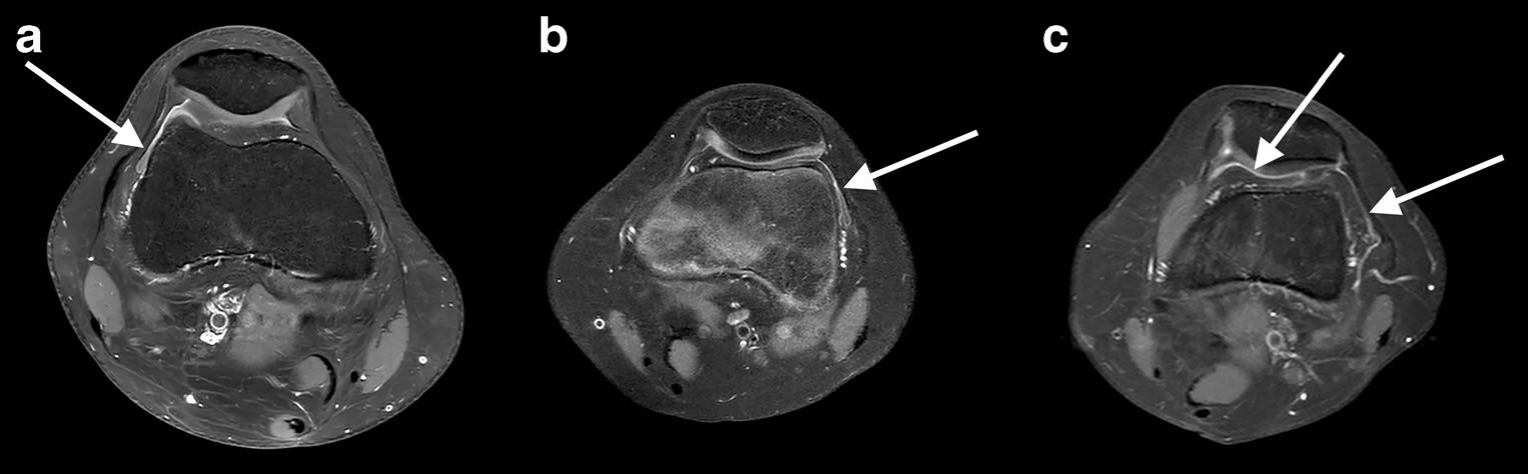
Normal enhancing synovial membrane in three different healthy children (arrows). Axial T1-weighted fat saturated images after the administration of an intravenous contrast agent
